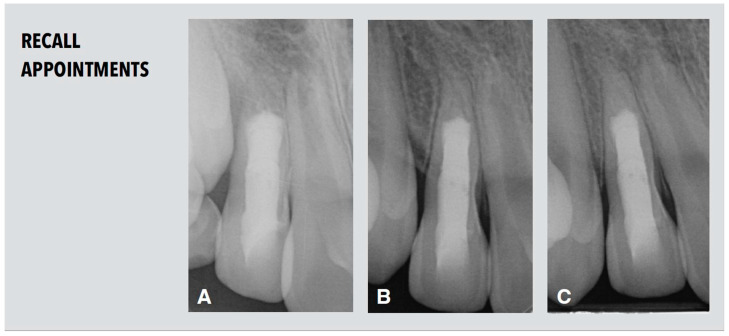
Radiological follow-up. (A) Periapical radiograph after nine months demonstrating increase in both root length and thickness in the apical part below the MTA level. (B,C) Periapical radiographs after two years (B) and after three years and nine month (C) demonstrating further root maturation.
